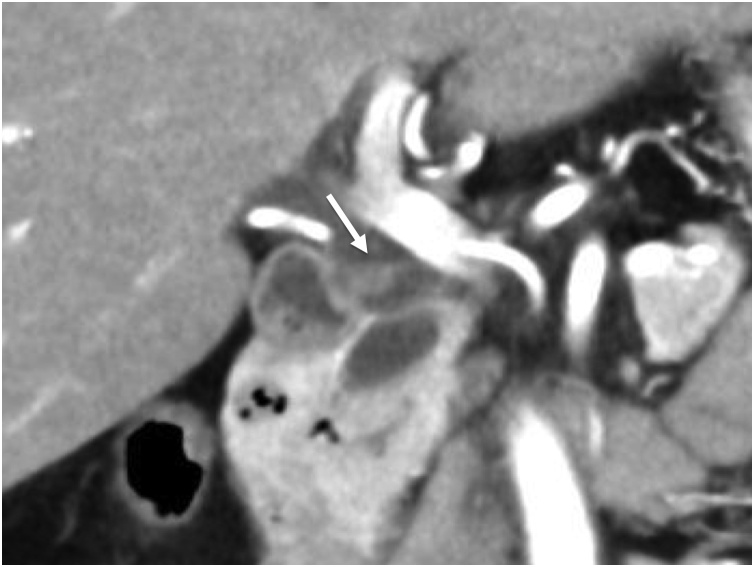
Enhanced computed tomography findings. Enhanced abdominal computed tomography showed the tumor (white arrow) adjacent to the common bile duct.
